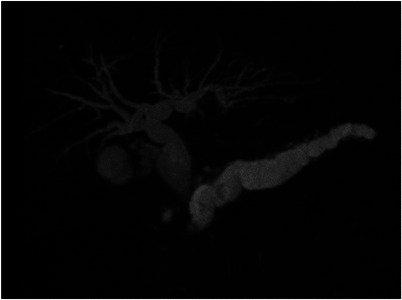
Magnetic resonance imaging performed when the patient initially visited our hospital 9 years ago. Intrapancreatic bile duct stricture, pancreatic duct stricture in the pancreatic head, and distal pancreatic duct dilation were observed.
